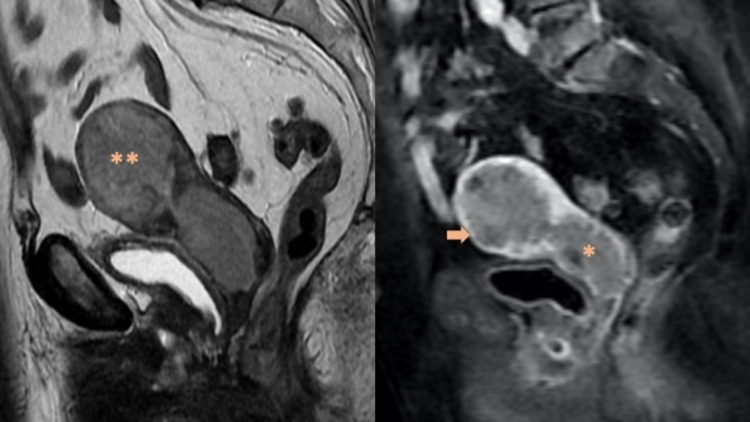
Stage II endometrioid adenocarcinoma in an 82-year-old woman. Sagittal T2-weighted MRI demonstrates intermediate to high-signal-intensity carcinoma () infiltrating the cervical stroma, which is better delineated after intravenous administration of contrast material (*). The tumor also invades the outer one-half of the myometrium (arrow).
